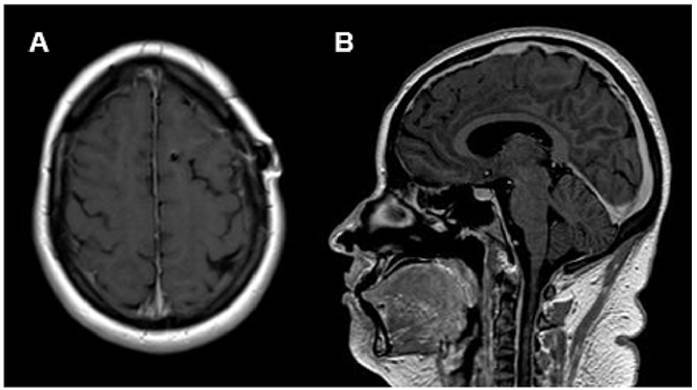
Axial (A) and sagittal (B) post-contrast T1-weighted images after the institution of immunosuppressive treatment. Note the absence of radiological evidence for leptomeningeal enhancement.
